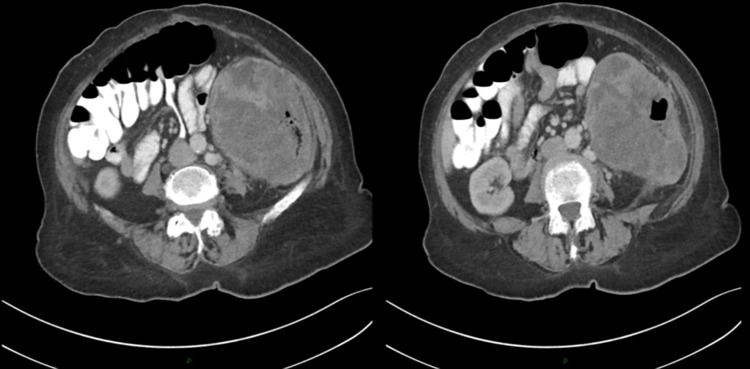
Computed tomography scan showing a large heterogeneous mass originated from the descending colon with surrounding multiple regional lymph nodes and fat stranding
